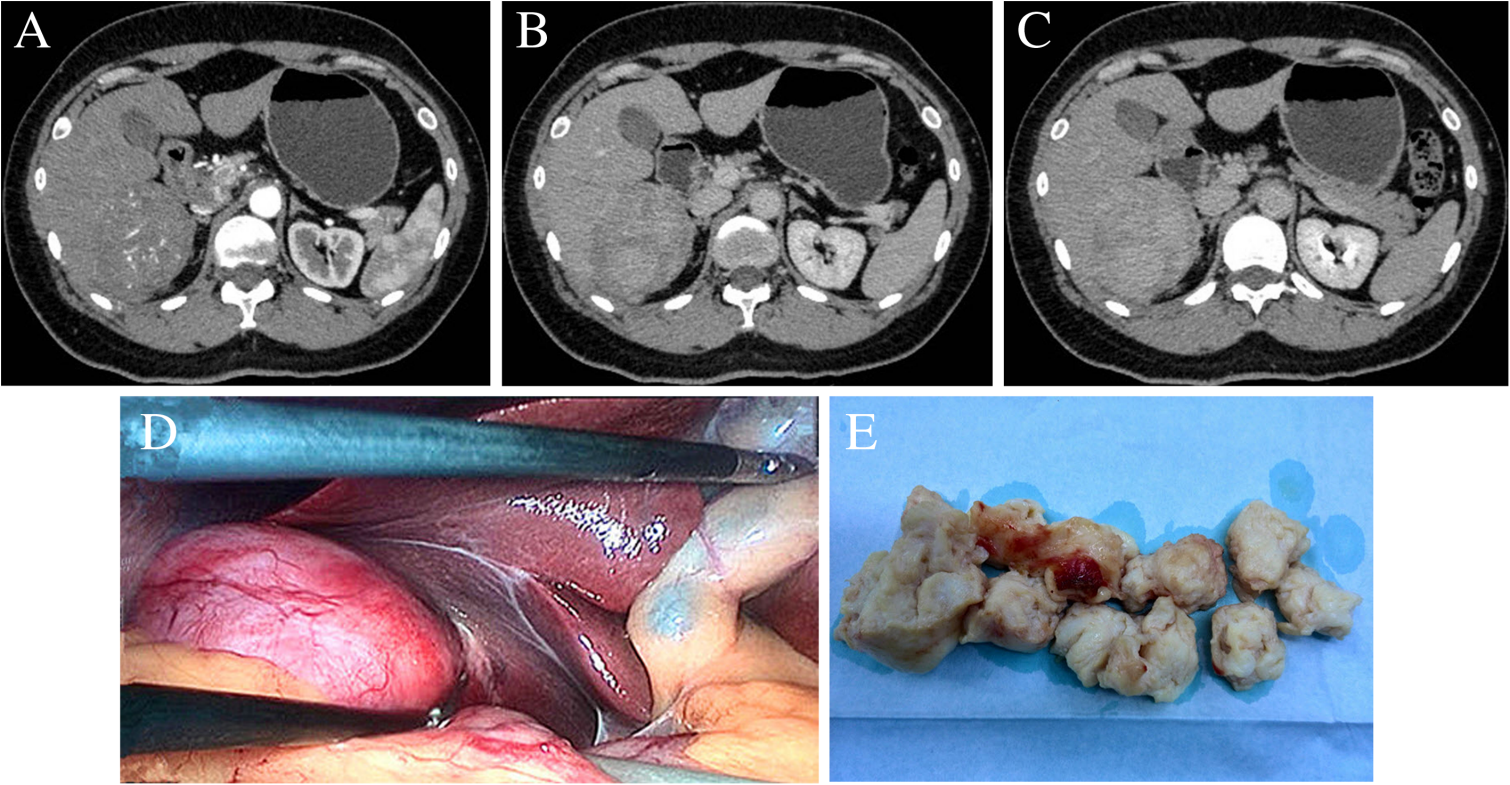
Abdominal CT scan images of the patients. CT images demonstrate (a) heterogenous enhancement in the artery phases; b progressive enhancement in the portal venous phases; and c prolonged enhancement in the balanced phases. d An image during the laparoscopic partial hepatectomy. e Tumor tissue samples
